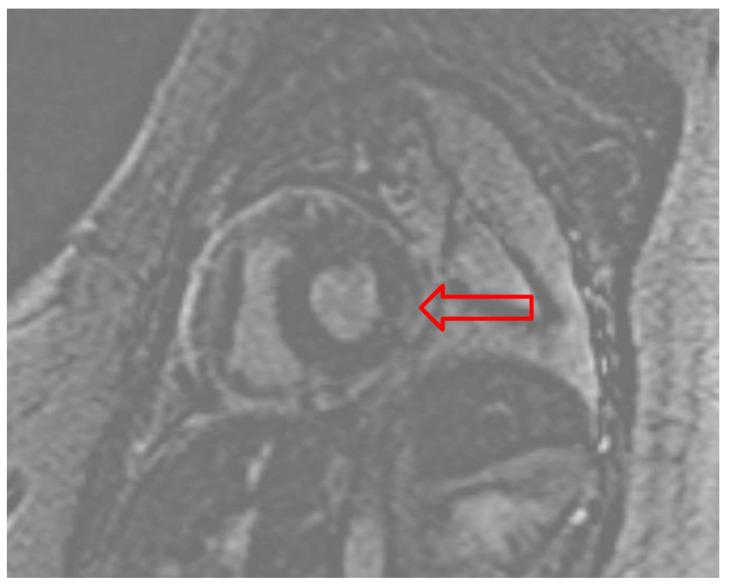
Gadolinium enhanced cardiac MRI pointing to the patchy sub-epicardial delayed enhancement of the basal lateral/inferolateral wall (red arrow).
